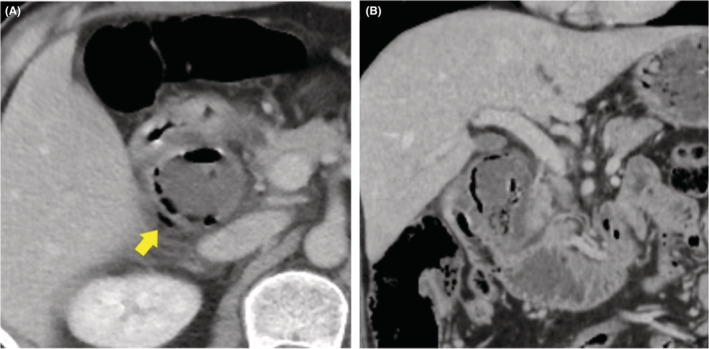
Abdominal computed tomography (CT) (A) axial image, (B) coronal image. CT revealed an enlarged duodenal diverticulum within the second portion of the duodenum and free air in the retroperitoneum (yellow closed arrow)
